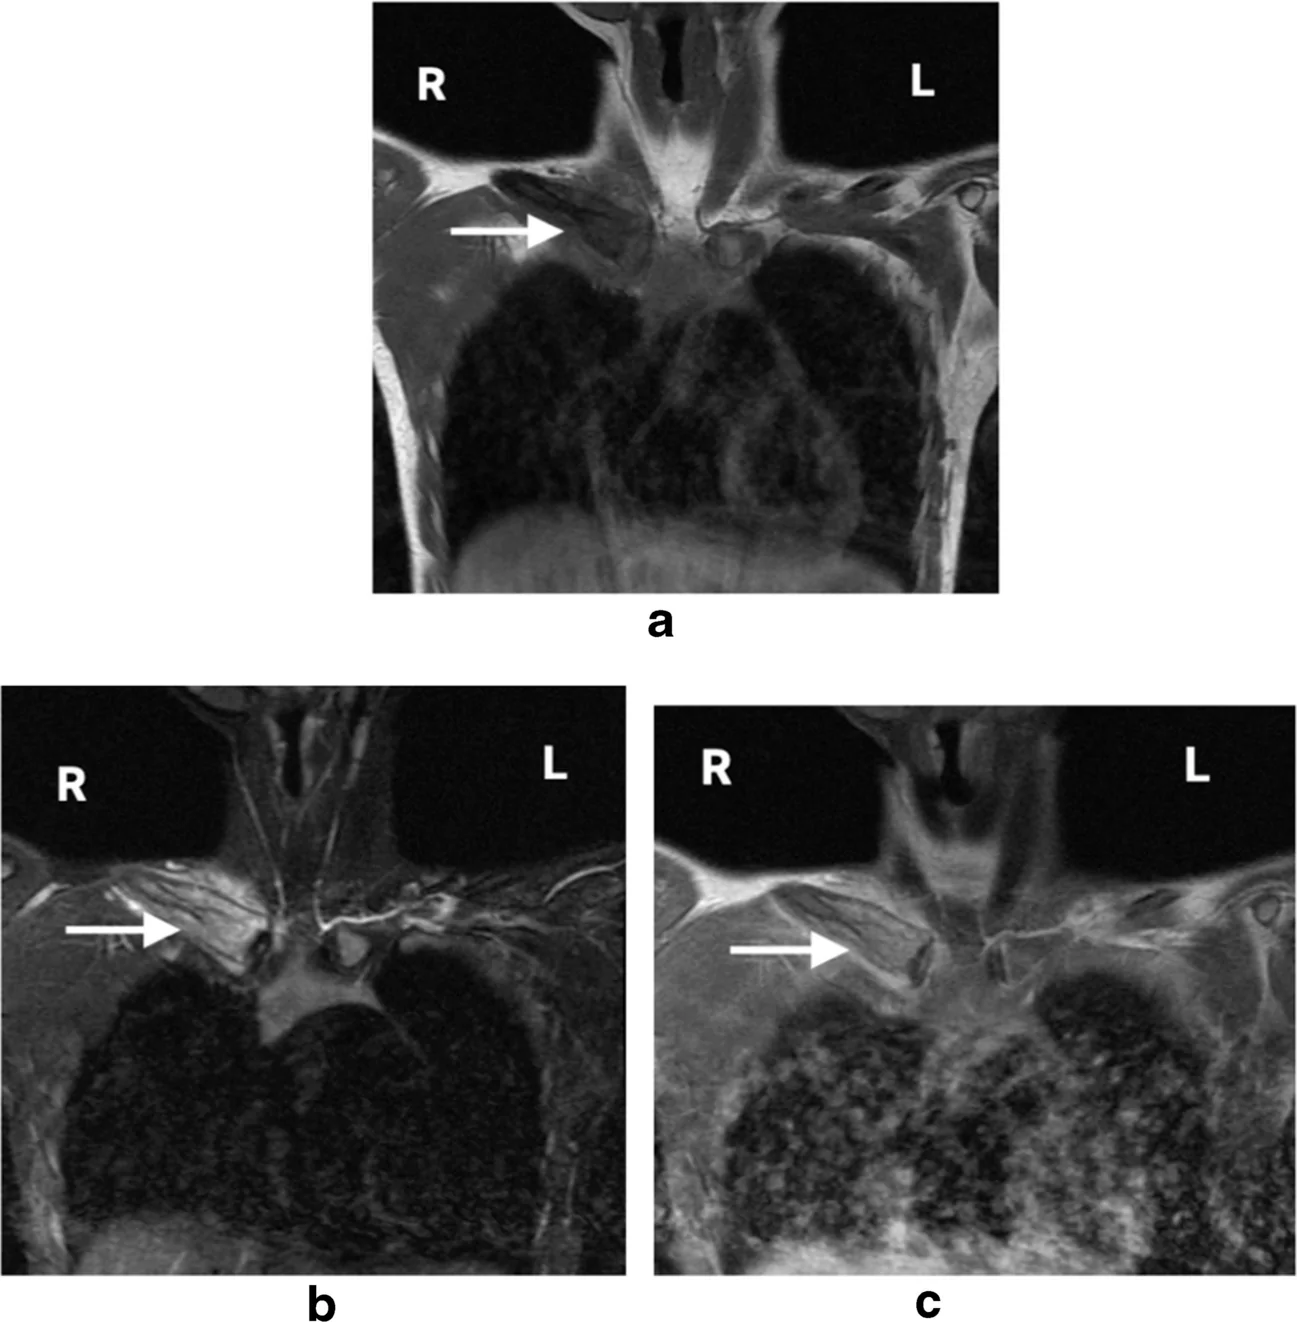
Images obtained of 10-year-old girl with CNO involving the right clavicle. a Coronal T1 weighted MR imaging of the right clavicle shows low signal intensity in the medial 2/3 of the right clavicle, extending laterally from the physis on T1 weighted images (arrow), with associated periosteal reaction that may appear prominent or aggressive. b Coronal STIR MR imaging of the right clavicle shows high signal intensity (arrow) (c) and enhances with gadolinium (arrow)
